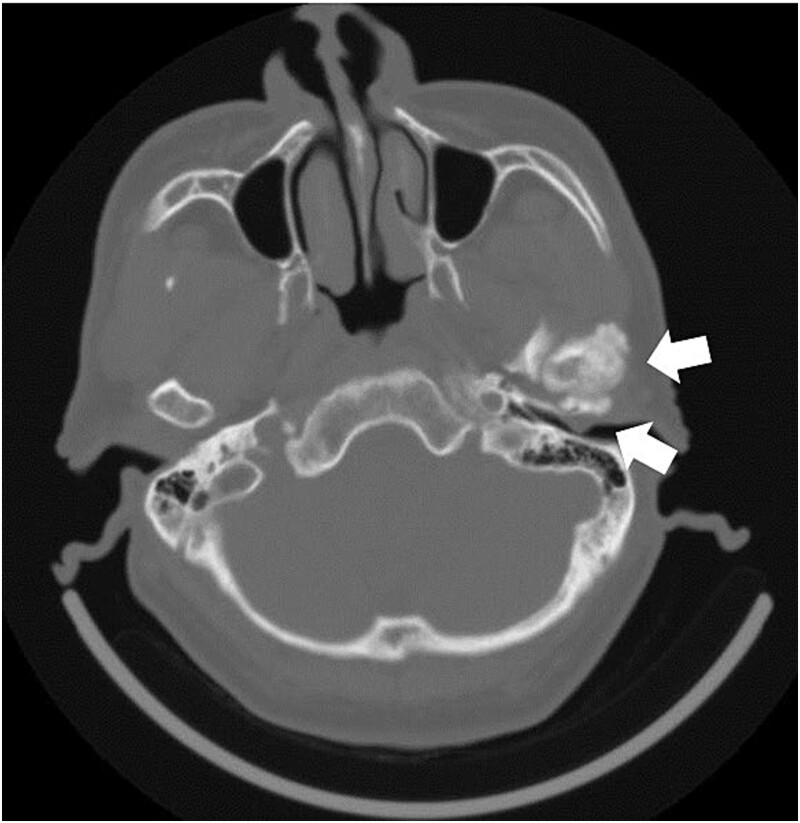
Axial section image of bone conditions on contrast-enhanced CT. CT image shows enlargement of the left temporomandibular head and a mass in the external auditory canal.
